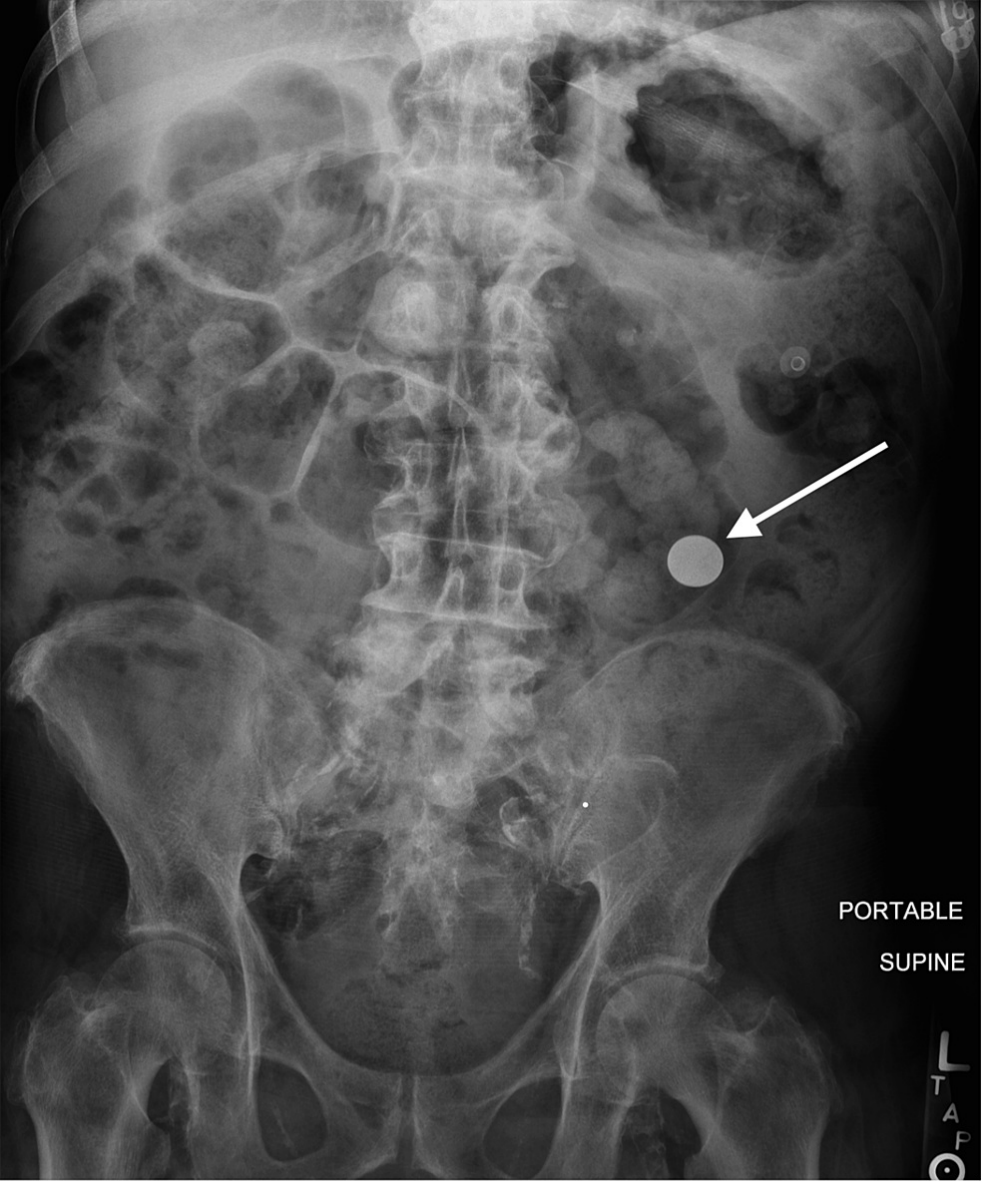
Abdominal X-ray after esophagogastroduodenoscopy (EGD)
